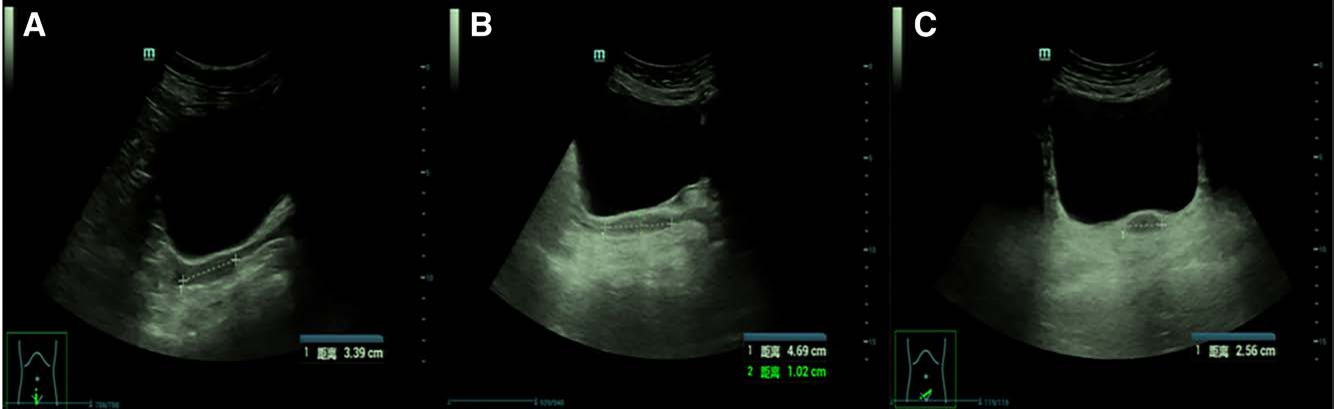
Uterine B-ultrasonography. The outline of the uterus is not clear; the size is about 34 × 10 × 26 mm. (A) An ultrasound image of the uterus with the measurement of the superoinferior diameter of the uterine body, which is 3.39 cm. (B) An ultrasound image showing multiple measurements. The distance from the uterine body to the cervix is measured as 4.69 cm, and the anteroposterior diameter of the uterine body is 1.02 cm. (C) An ultrasound image of the uterus with the measurement of the transverse diameter of the uterine body, which is 2.56 cm.
